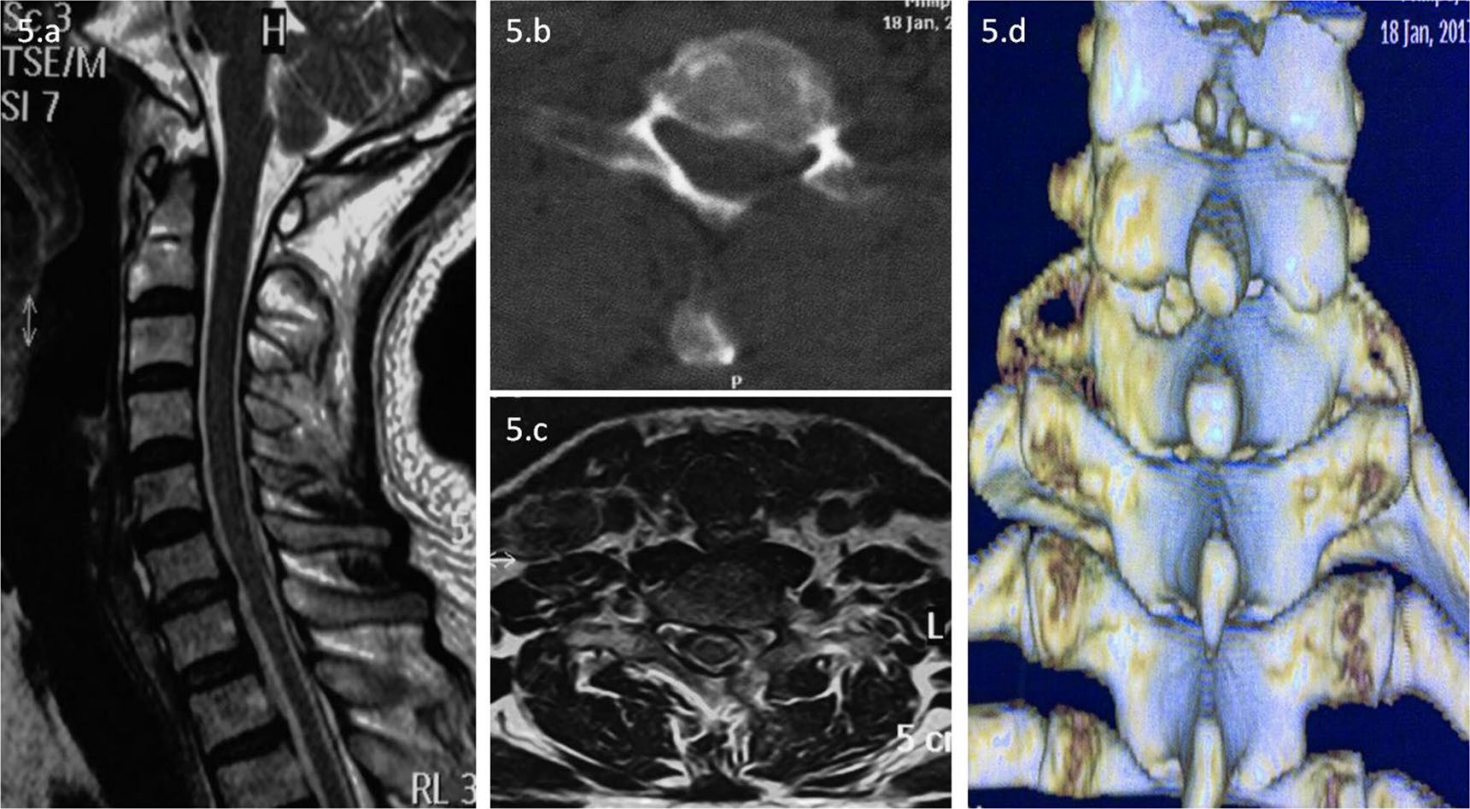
Postoperative MRI and CT. Sagittal and axial MRI (a, c) showed no disc herniation pressing on the spinal cord or nerves. Axial CT (b) showed no disc herniation impairing the spinal cord or nerves. The 3D reconstruction image (d) shows the bone structure removed by the Delta technique.
